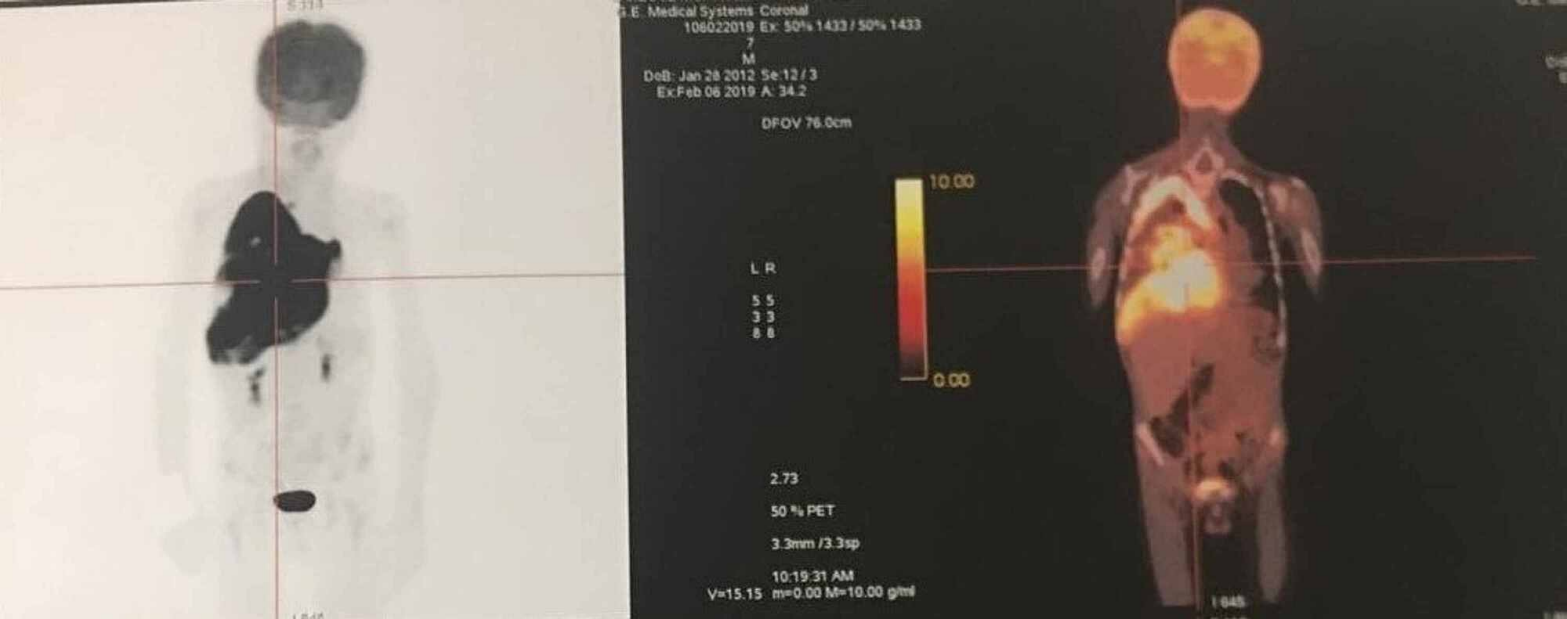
The positron emission tomography/computed tomography (PET/CT) detects a hyper-metabolic zone of the entire right hemitorax.
